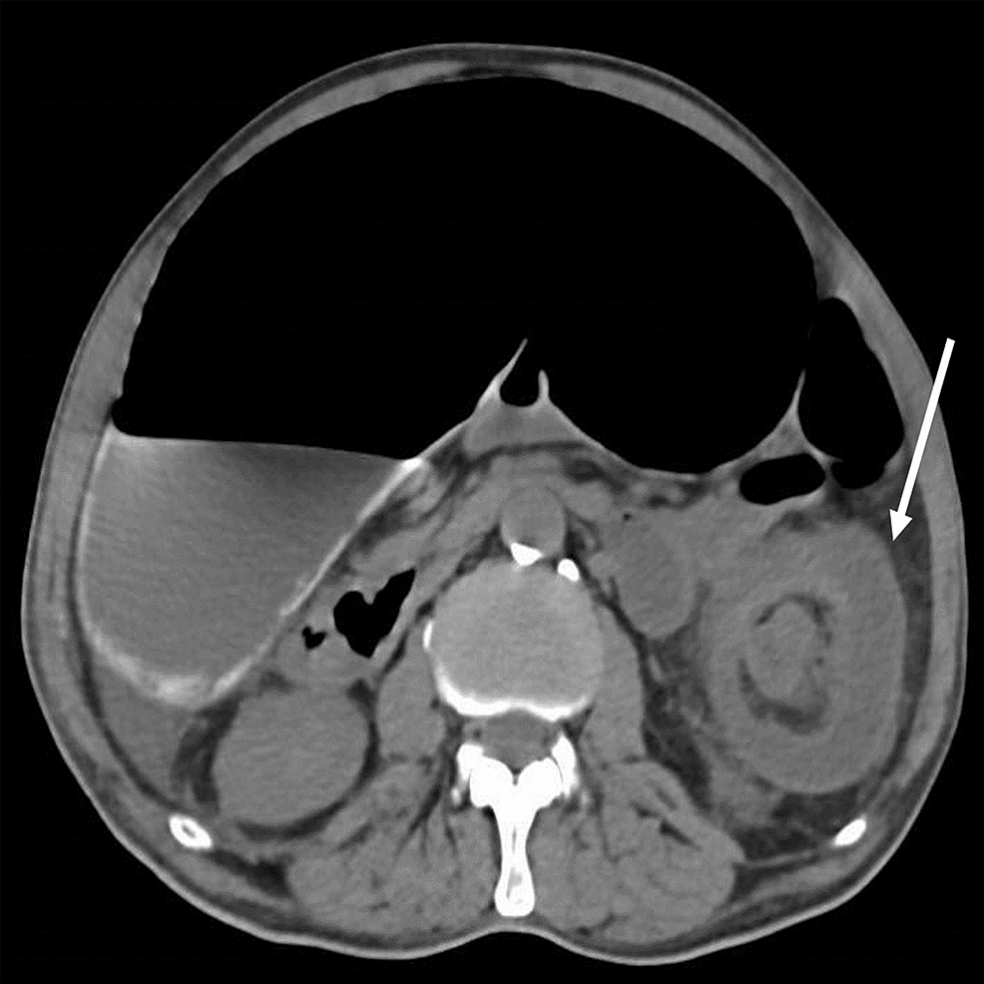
Abdominal CT scan showing a classic target sign, suggestive of a colocolic intussusception
